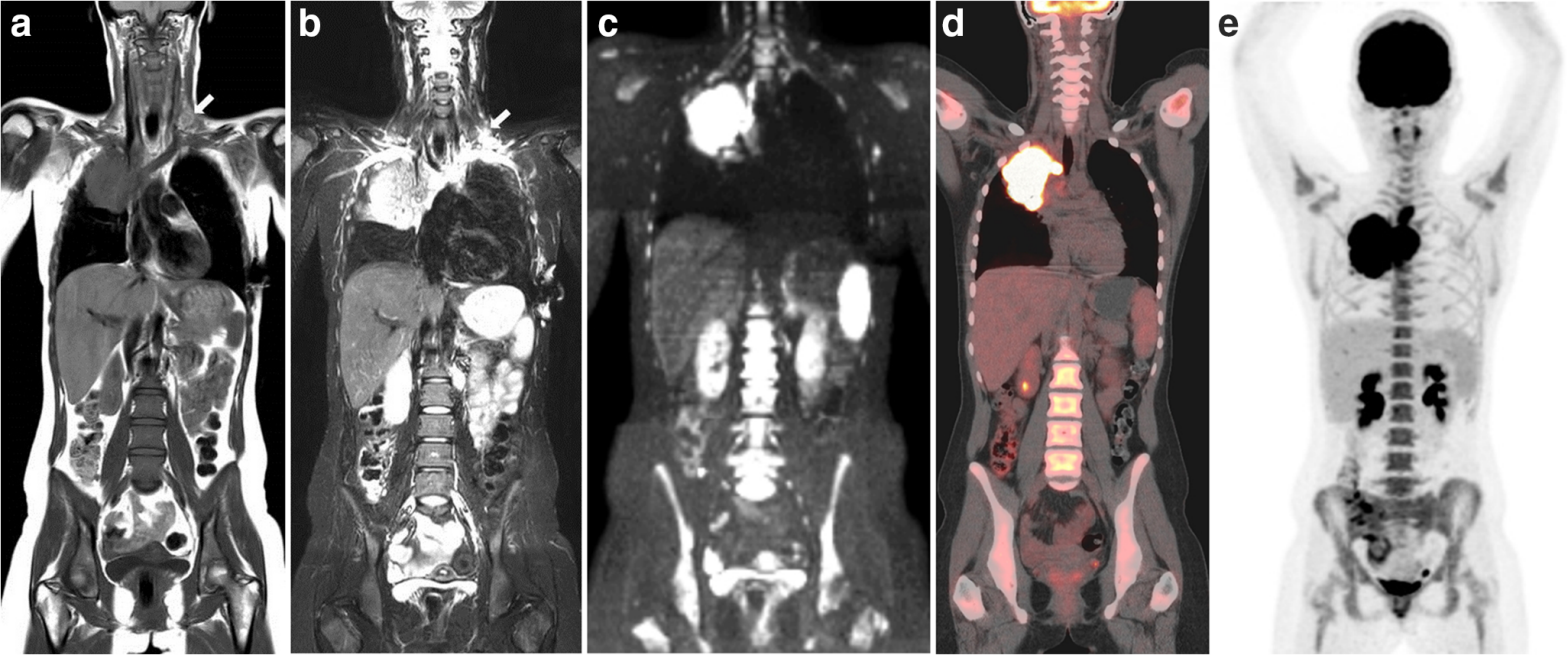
A 17-year-old girl with stage IIE Hodgkin lymphoma. Example of an interpretation error by the WB-MRI readers due to a lack of clinical data. A lesion due to the placement of a central venous catheter was mistaken for presence of Hodgkin lymphoma (arrows). Furthermore, an E-lesion in the lung originating from the mediastinum is present. Coronal T1-weighted (a) and T2-weighted (b) MRI, high *b* value DWI (b800) (c), coronal FDG-PET/CT (d) and coronal maximum intensity projection (MIP) of the FDG-PET/CT (e) are shown. Note that the supraclavicular lesion was not seen at the FDG-PET/CT MIP (e)
